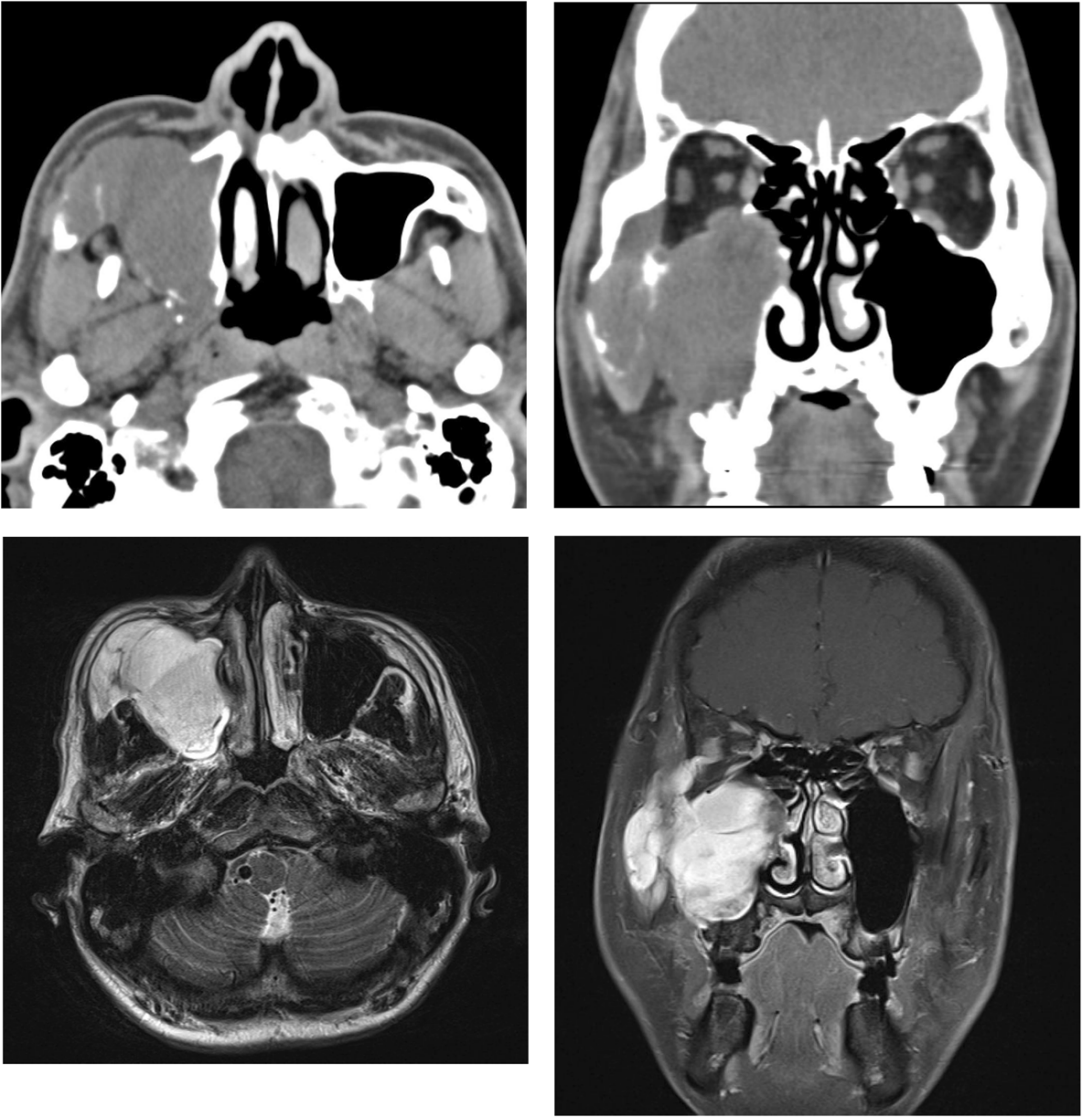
Paranasal CT and MRI findings of maxillary sinus MFH. The figures show a large mass involving the anterior, posterolateral, and medial walls of the right maxillary sinus; inferior and lateral walls of the right orbit; anterior aspect of the right zygomatic arch; right pterygoid bone; and anterior surface of the right sphenoid bone.
